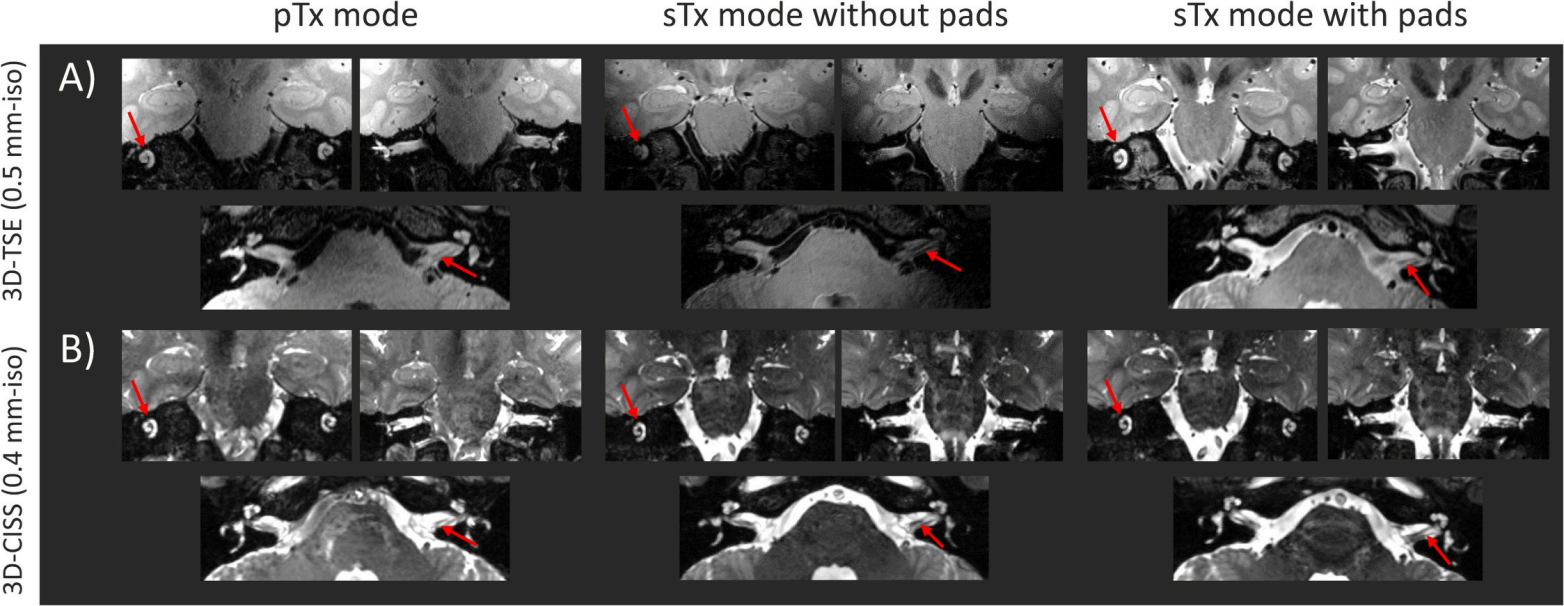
High-resolution MR images of the inner-ears, given for the coil models shown in Fig 1. For parallel-transmit (pTx mode), and single transmit modes with and without dielectric pads (sTx mode without/with pads, respectively): A) high-resolution 3D-TSE (0.5 mm-isotropic) and B) high-resolution 3D-CISS (0.4 mm-isotropic) images shown in two coronal and one transverse slice. The red arrows indicate the structures of interest, namely the cochlea and the auditory nerves. The MR images are shown for a same subject.
